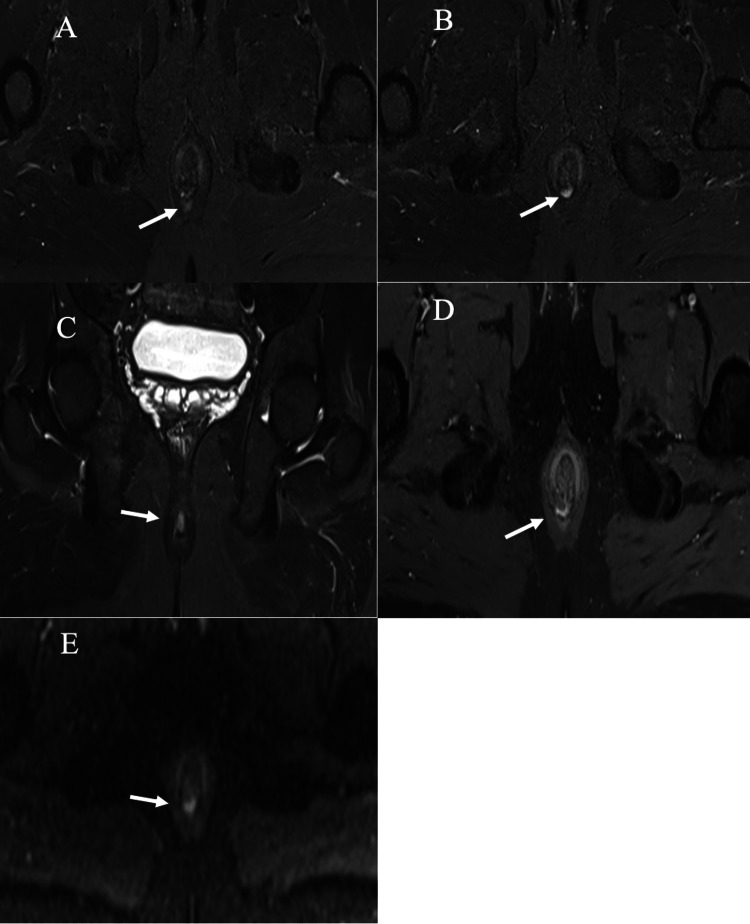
Grade 1 simple linear intersphincteric fistula: (A-B) Axial STIR images showing a simple linear intersphincteric fistula (A) with an internal opening at the 6 o’clock position (B). (C-D) Coronal STIR and axial post-contrast-enhanced fat-suppressed T1 images showing a simple linear intersphincteric fistula. (E) Axial DWI showing restricted diffusion on DWI. STIR: short tau inversion recovery; DWI: diffusion-weighted imaging
